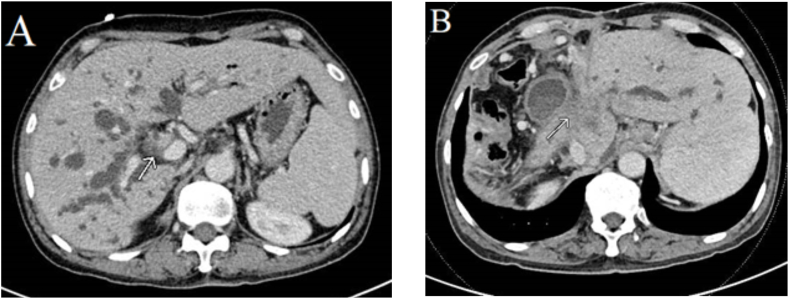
A 60-year-old man with perihilar cholangiocarcinoma with periductal fat invasion (white arrow; Bismuth-Corellete 3A) on pre-operative imaging (Fig. 7A). Post-curative resection follow-up CT at 13 months shows infiltration of a recurrent tumor at the surgical bed (white arrow; Fig. 7B).
